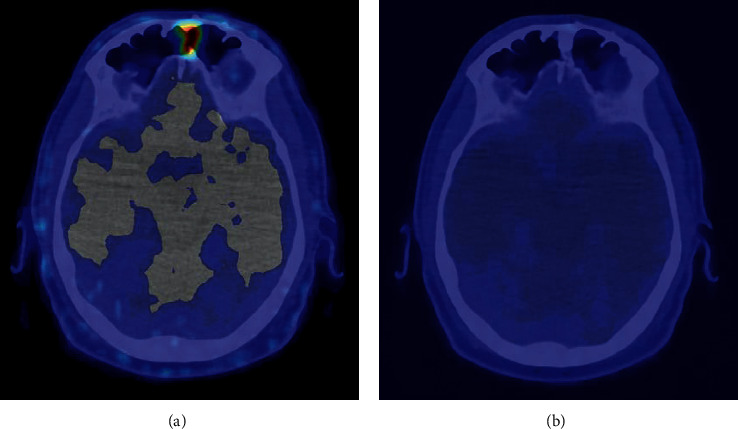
^68^Ga-DOTATATE PET-CT images showing complete response of the recurrent ossifying fibromyxoid tumor in the left frontal sinus after surgery and adjuvant radiotherapy. ^68^Ga-DOTATATE PET-CT images before treatment (a) and after treatment (b).
